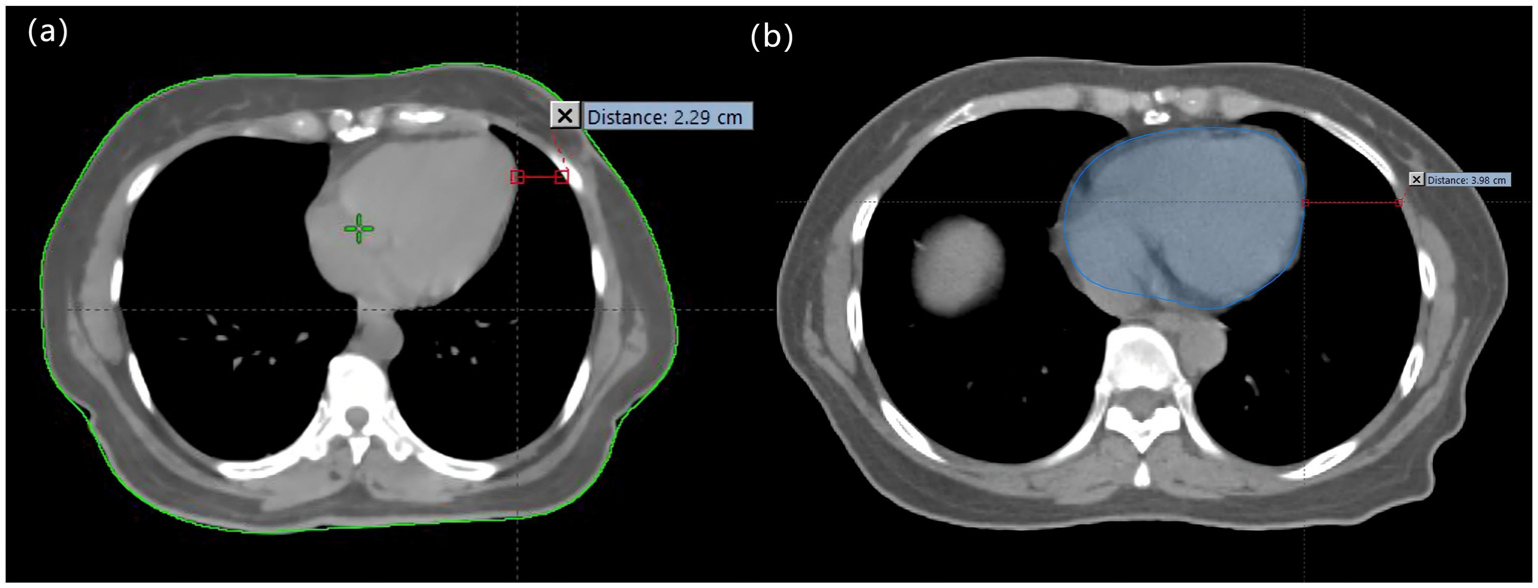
Measurement of the HCD on CT transverse imaging (A) and coronary imaging (B).
